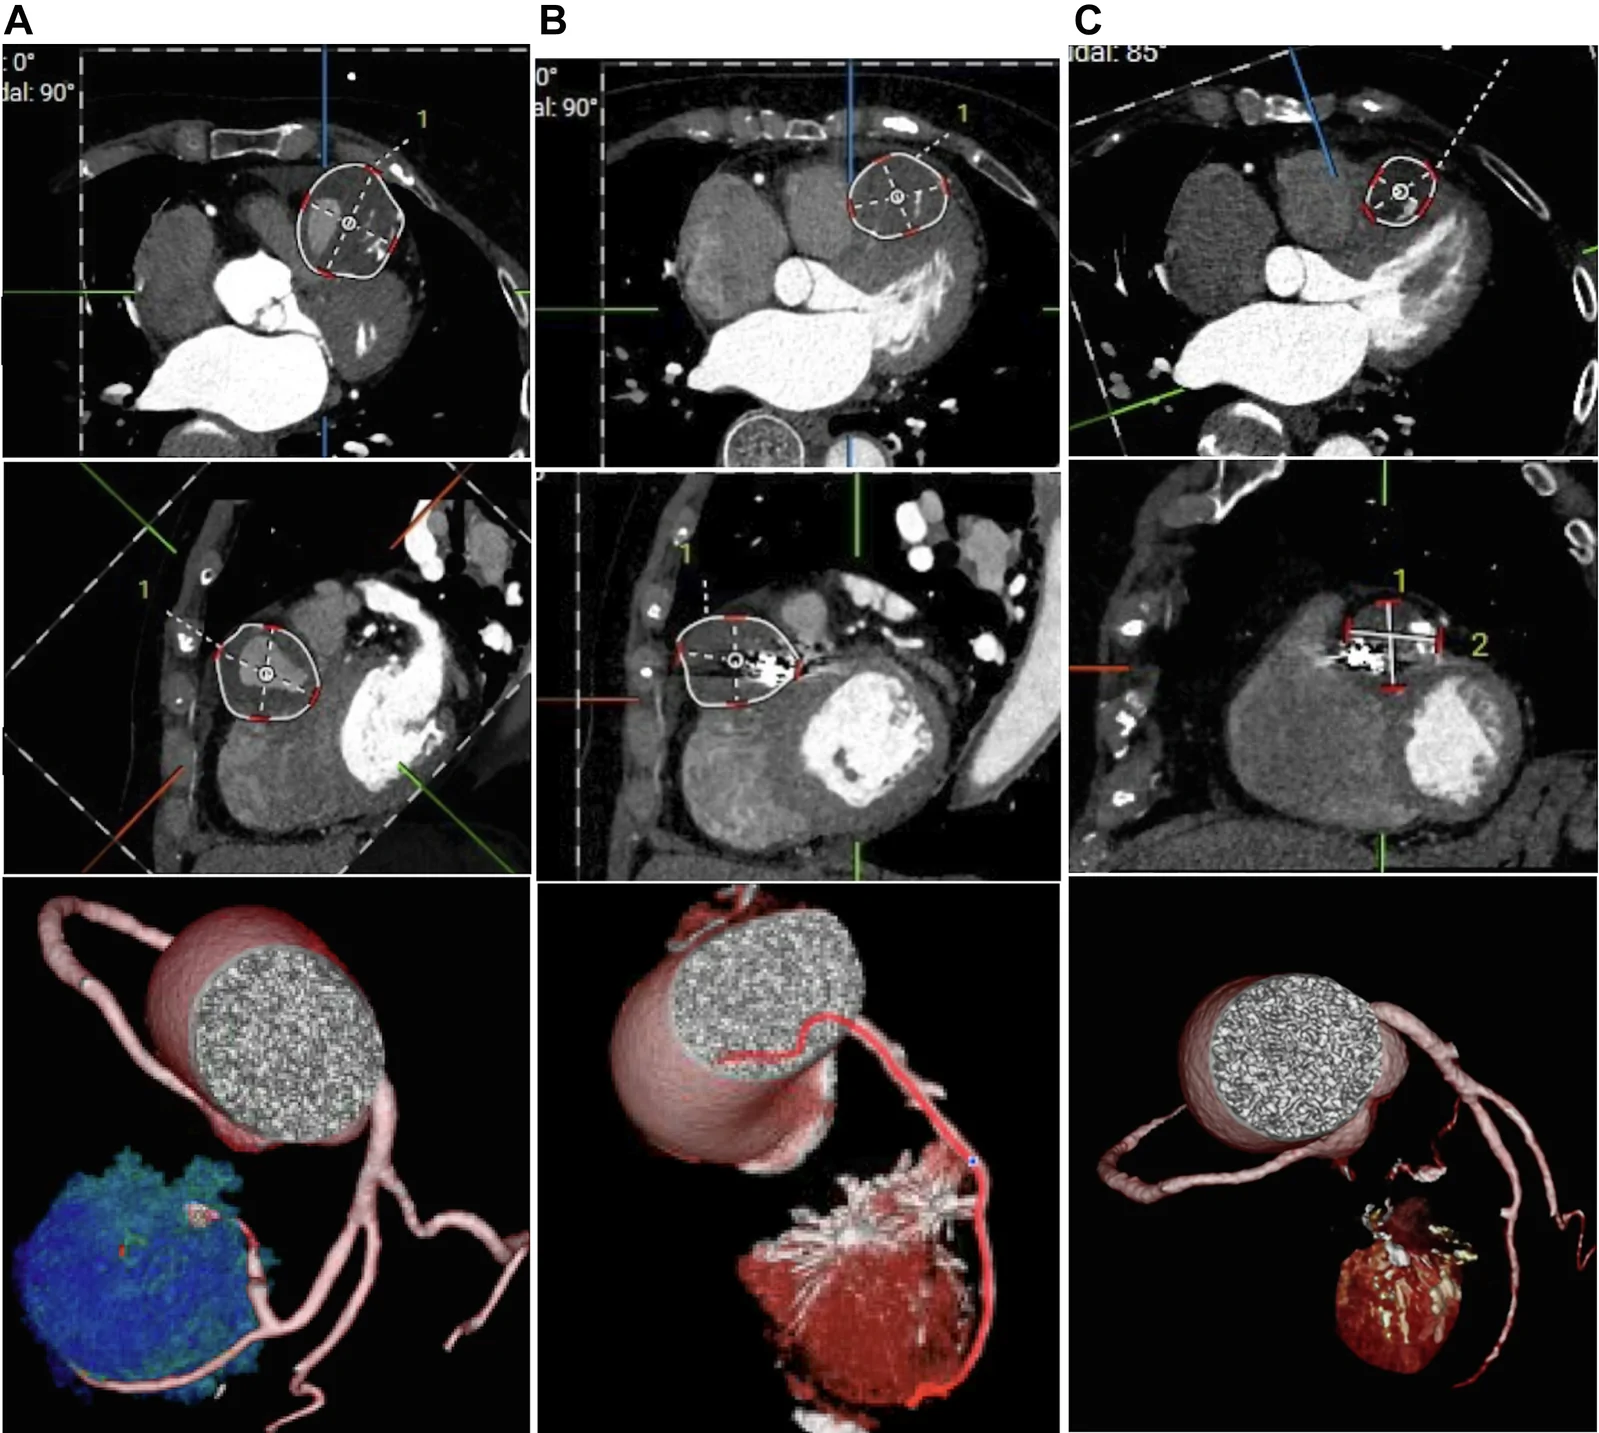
Computed Tomography and Three-Dimensional Reconstruction of Coronary Aneurysm at Baseline and Follow-Up Initial computed tomography imaging and 3-dimensional rendering of the aneurysm at baseline (A) and follow-up imaging at 1 month (B) and 6 months (C). Measurements were obtained at the largest aneurysm dimensions identified in each imaging plane. (A) Right anterior oblique caudal view 46 × 40 mm; left anterior oblique caudal view 42 × 36 mm. (B) Right anterior oblique caudal view 39 × 32 mm; left anterior oblique caudal view 43 × 30 mm. (C) Right anterior oblique caudal view 30 × 22 mm; left anterior oblique caudal view 31 × 32 mm showing aneurysm is completely thrombosed.
